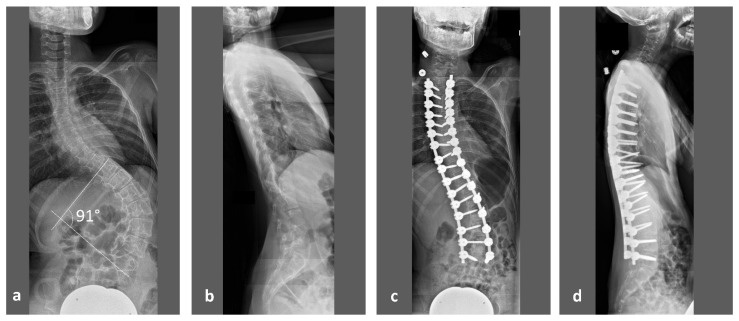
13-years-old, non-ambulatory girl with RTT with severe neuromuscular scoliosis and thoracic hyper-kyphosis. (a) Preoperative anteroposterior (ap) and (b) lateral sitting radiographs with a scoliosis angle of 91°. (c) Postoperative ap and (d) lateral sitting radiographs with dorsal spinal fusion from T (thoracic) 2 to L (lumbar) 5.
